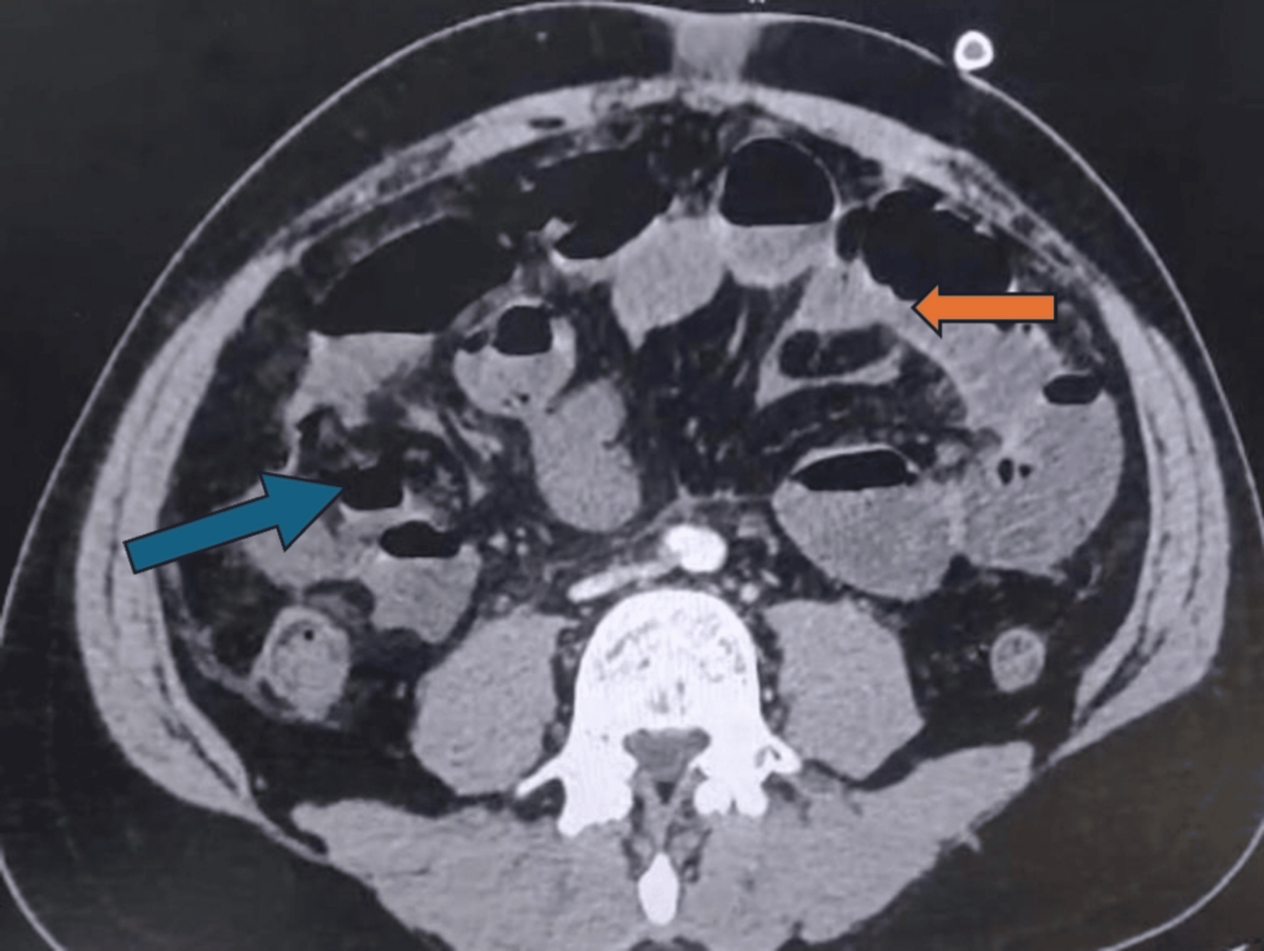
Axial contrast-enhanced computed tomography (CECT) image of the abdomen demonstrating bowel ischemia and pneumatosis The orange arrow indicates a segment of hypoenhancing bowel loop consistent with non-viable ischemic bowel. The blue arrow demonstrates intramural air within the bowel wall (pneumatosis intestinalis), indicative of transmural necrosis and impending perforation.
